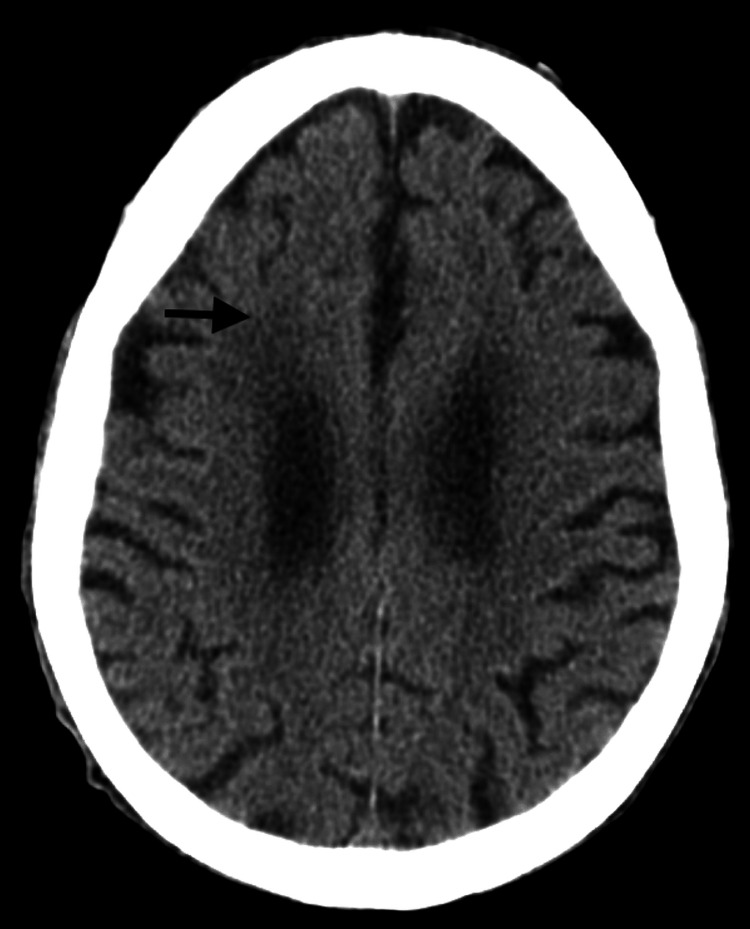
Non-contrast cranial computed tomography demonstrating ischemic leukoencephalopathy (arrow)
